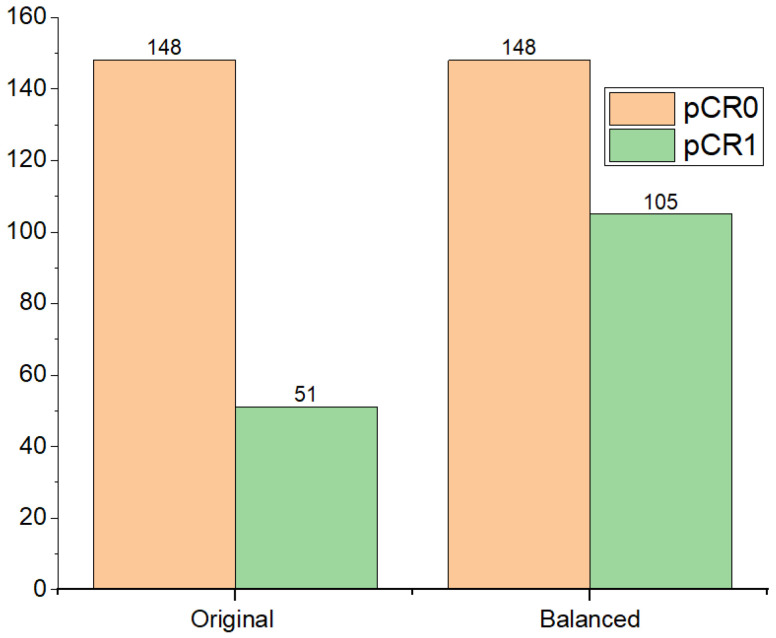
Data sample distribution in pCR0 and pCR1 classes of ISPY-1. In the original dataset, each patient was represented by a single instance of MRI scans. However, in the balanced dataset, we have made updates to include multiple MRI scans at the same time-stamp for patients who responded to the NST.
